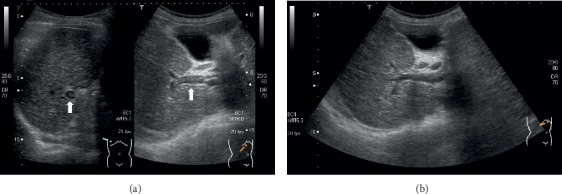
Ultrasonogram of the liver. (a) Before treatment: portal thrombus was seen in the right portal branch (arrow). (b) The thrombus was almost completely dissolved on day 6.
